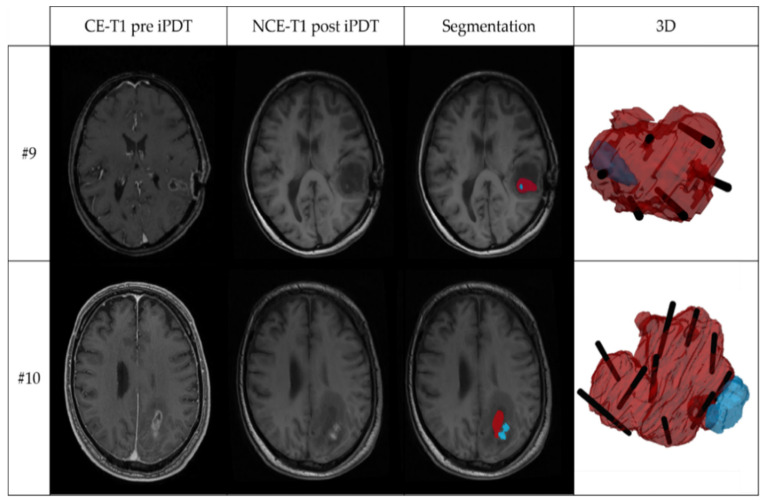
Two examples of MRI and 3D superimposition visualization, showing: contrast-enhanced T1-weighted MRI before iPDT (CE-T1 pre iPDT); non-contrast-enhanced T1-weighted MRI after iPDT (NCE-T1 post iPDT); segmentation of the tumor volume (red) and intrinsic T1 hyperintensity (blue) based on the CE-T1 pre iPDT and NCE-T1 post iPDT images; and 3D superimposition of tumor volume (red), intrinsic T1-hyperintensity volume (blue), and planned localization of irradiating tips of cylindrical diffuser fibers (black). The upper panel shows iPDT case #9 with the largest overlap coefficient (OV = 0.99); the lower panel iPDT case #10 with smallest overlap coefficient (OV = 0.02).
